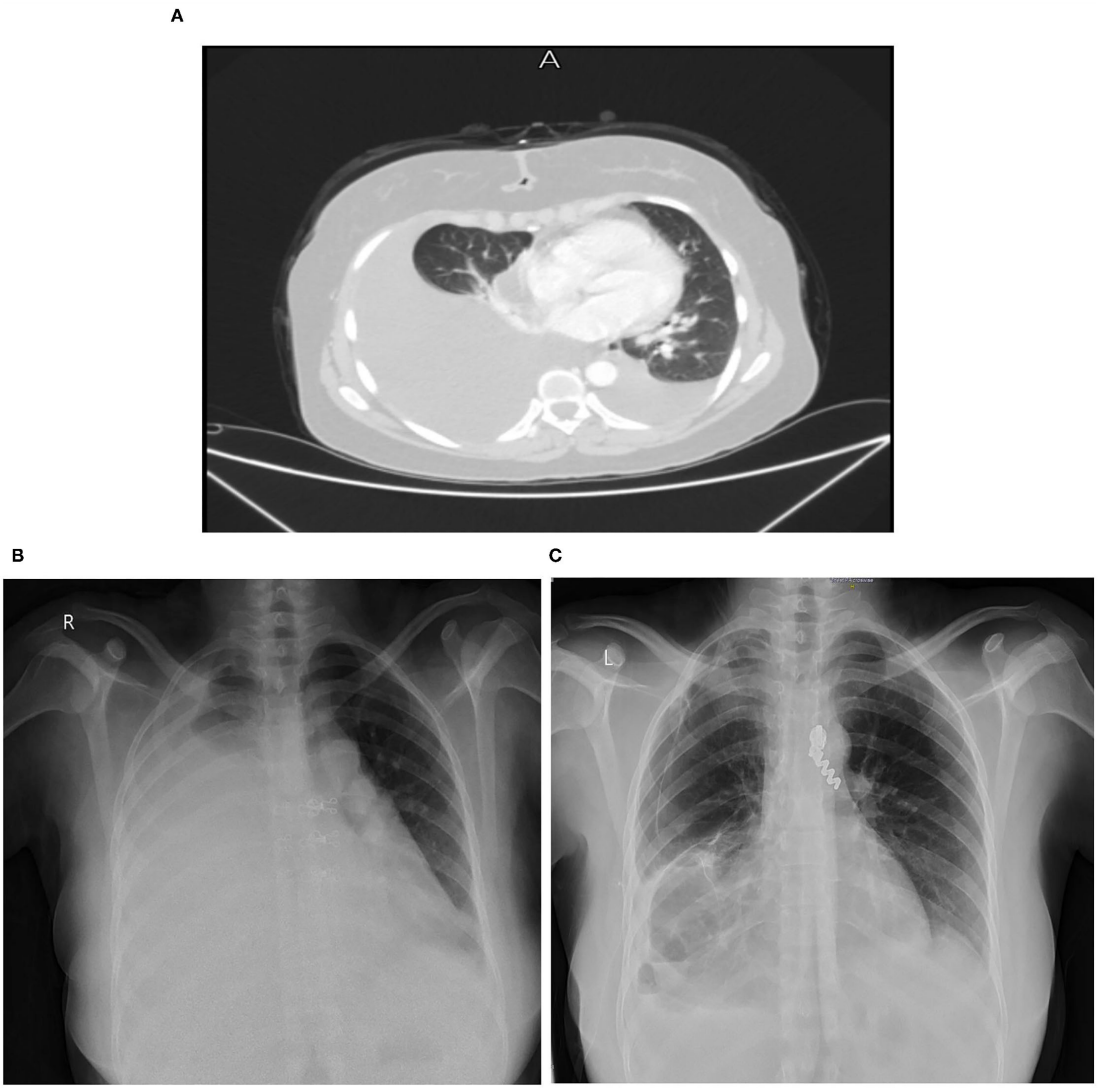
(A) Chest CT scan on August 17, 2020. (B) Chest X-ray on December 14, 2020. (C) Chest X-ray on April 17, 2021.
